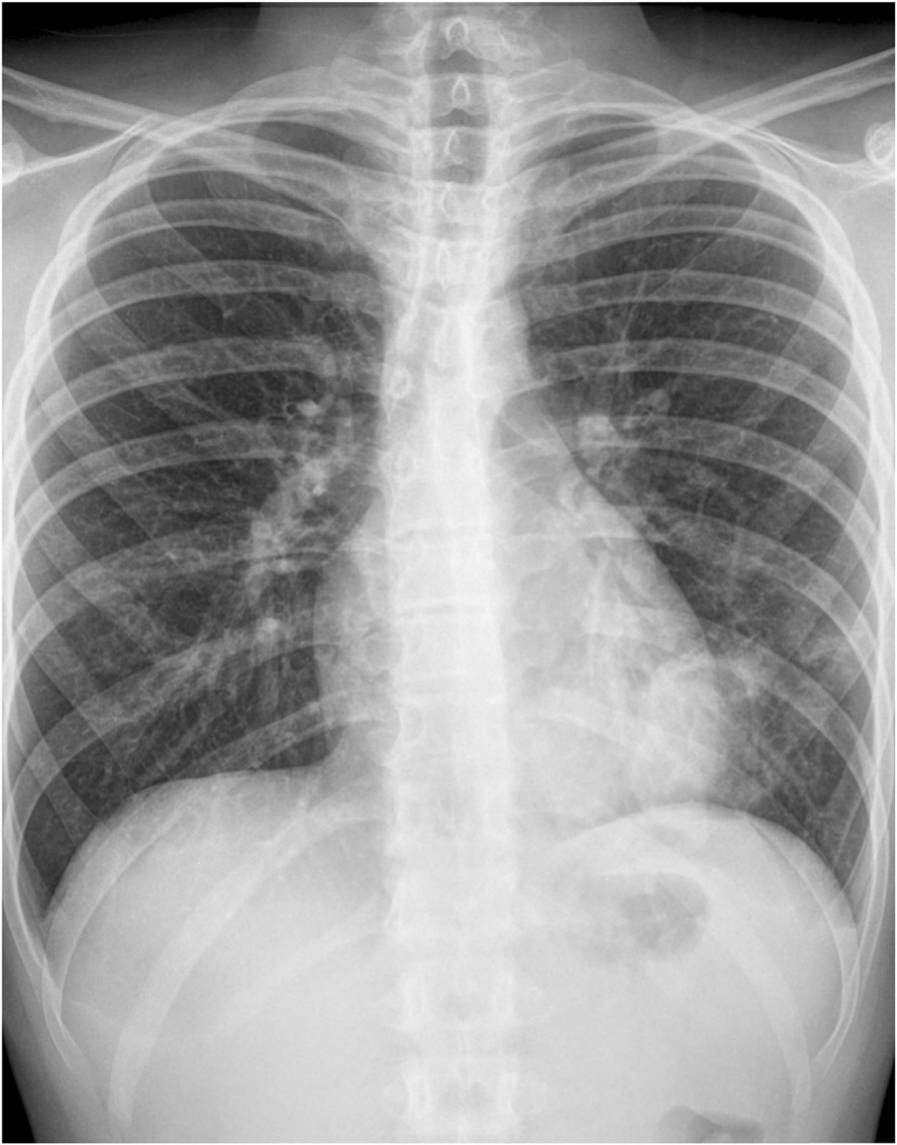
Frontal chest x-ray performed on day 14 showing an improvement of the opacity
